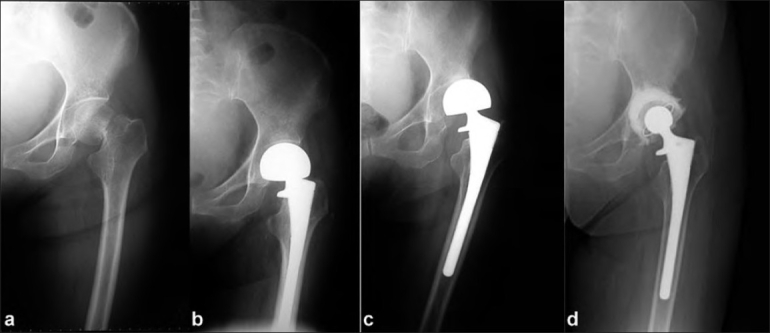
(a) An anteroposterior radiograph of left hip showing a femoral neck fracture and a radiologically normal acetabulum at the time of the injury (b) Immediate postoperative anteroposterior radiograph of the left hip showing no subluxation, (c) 9 months postoperative anteroposterior radiograph showing lateral migration of outer head. (d) Two years postoperative anteroposterior radiograph after conversion to total hip arthroplasty showing no signs of loosening or change in the position of the implant
